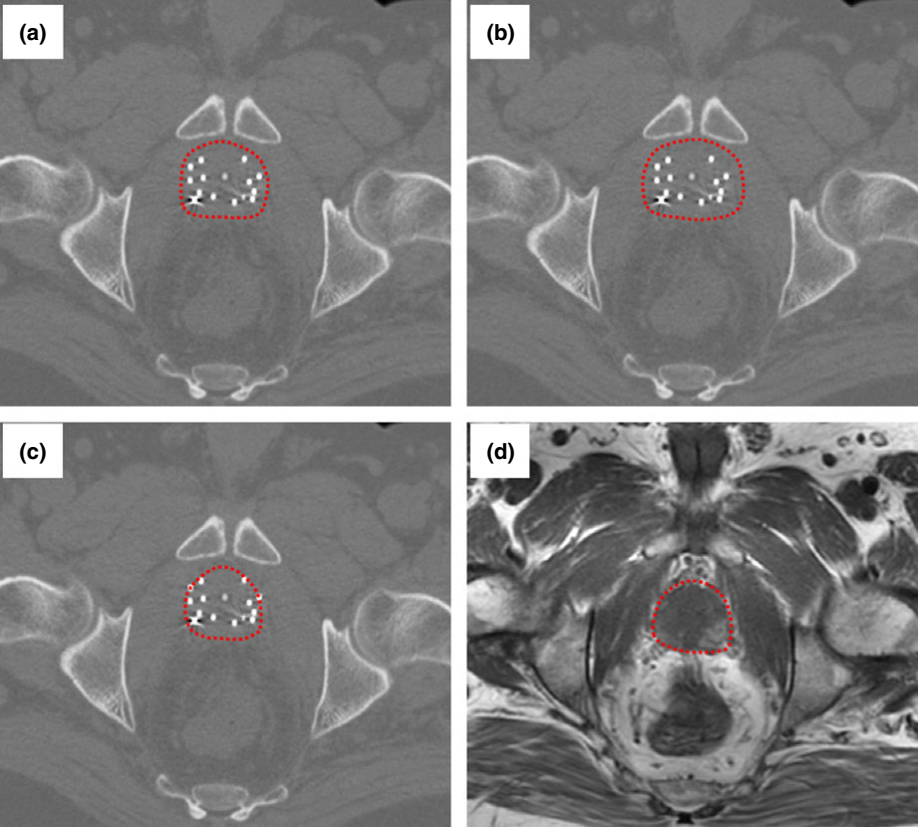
Inter‐observer CT‐based and TCDR‐based prostate contours: (a) physician 1 CT‐based, (b) physician 2 CT‐based, (c) TCDR‐based, and (d) MRI‐defined prostate contour (gold standard). The CT‐based contours (a) and (b) overestimate the prostate, and the TCDR‐based prostate contour (c) matches closely to the gold standard (d).
